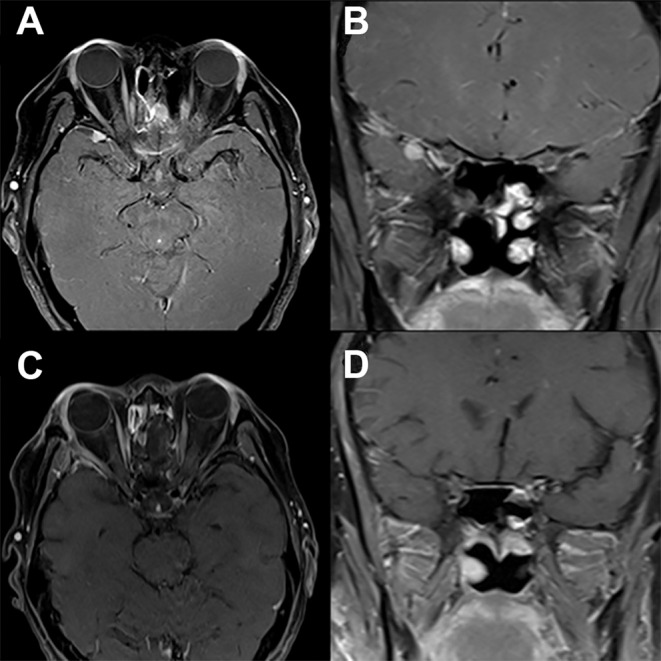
Modified lateral orbitotomy. Preoperative T1-weighted axial (A) and coronal (B) gadolinium-enhanced MRI in a patient with previous resection of esthesioneuroblastoma 5 years earlier demonstrate a new homogenously enhancing lesion along the right sphenoid wing. The location and small size of the lesion allows for complete removal via a right modified lateral orbitotomy orbitocranial approach. Postoperative T1-weighted axial (C) and coronal (D) gadolinium-enhanced MRI demonstrate complete resection of this lesion associated with full recovery and no new neurological findings.
